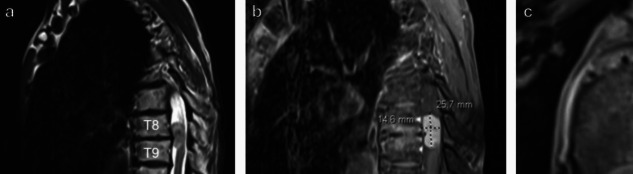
a Sagittal T2, b sagittal T1 post-contrast, and c axial T1 post-contrast MRI of the thoracic spine demonstrating the homogeneously enhancing lesion at T8 and T9 with severe spinal cord compression and displacement to the left
